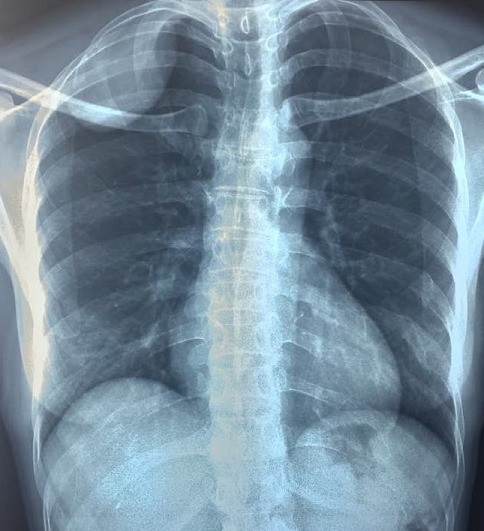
Une radiographie thoracique de face qui montre une opacité à projection apicale droite homogène bien limitée d'allure pariétale thoracique
